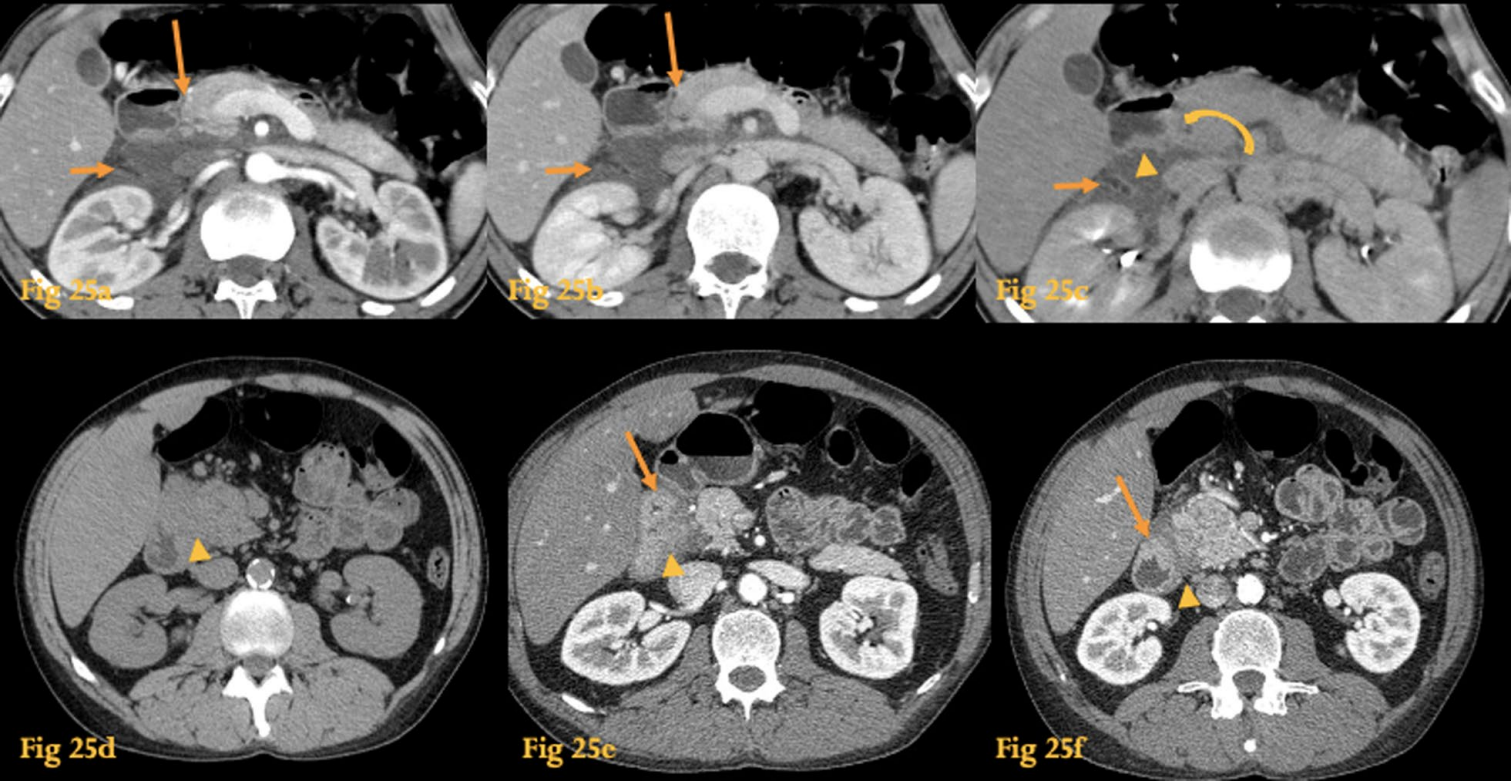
Paraduodenal pancreatitis. Abdominal CT of a patient with clinical and laboratory findings suggestive of pancreatitis (a–c). The pancreas has normal volume, morphology, and texture. There is retroperitoneal fluid in the right anterior pararenal space (small arrows) and in the pancreatoduodenal groove (big arrows). The posterior wall of the descending duodenum is slightly thickened (arrowhead) and the pancreatoduodenal groove reveals discrete late enhancement (curved arrow on c), suggestive of fibrotic changes. Abdominal CT of another patient with paraduodenal pancreatitis (d–f) shows an ill-defined soft-tissue mass within the groove (arrowheads) and descending duodenal medial wall thickening (arrows)
